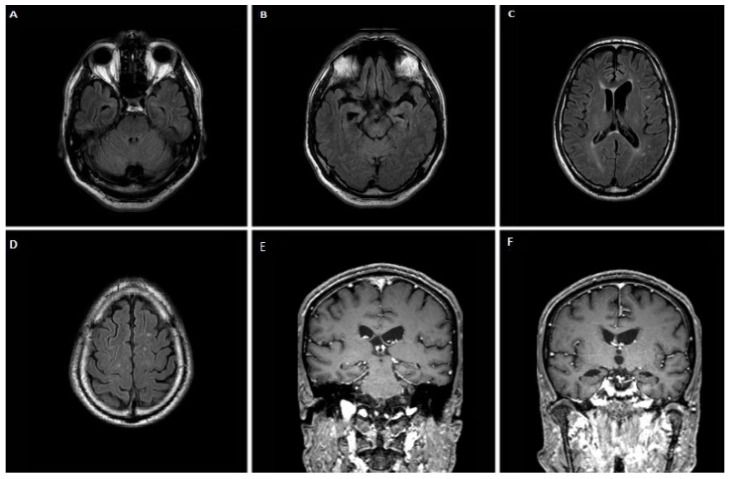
Brain MRI of case 5. Axial FLAIR images (A–D) and coronal T1 images (E,F) reveal mild frontal lobe atrophy.
